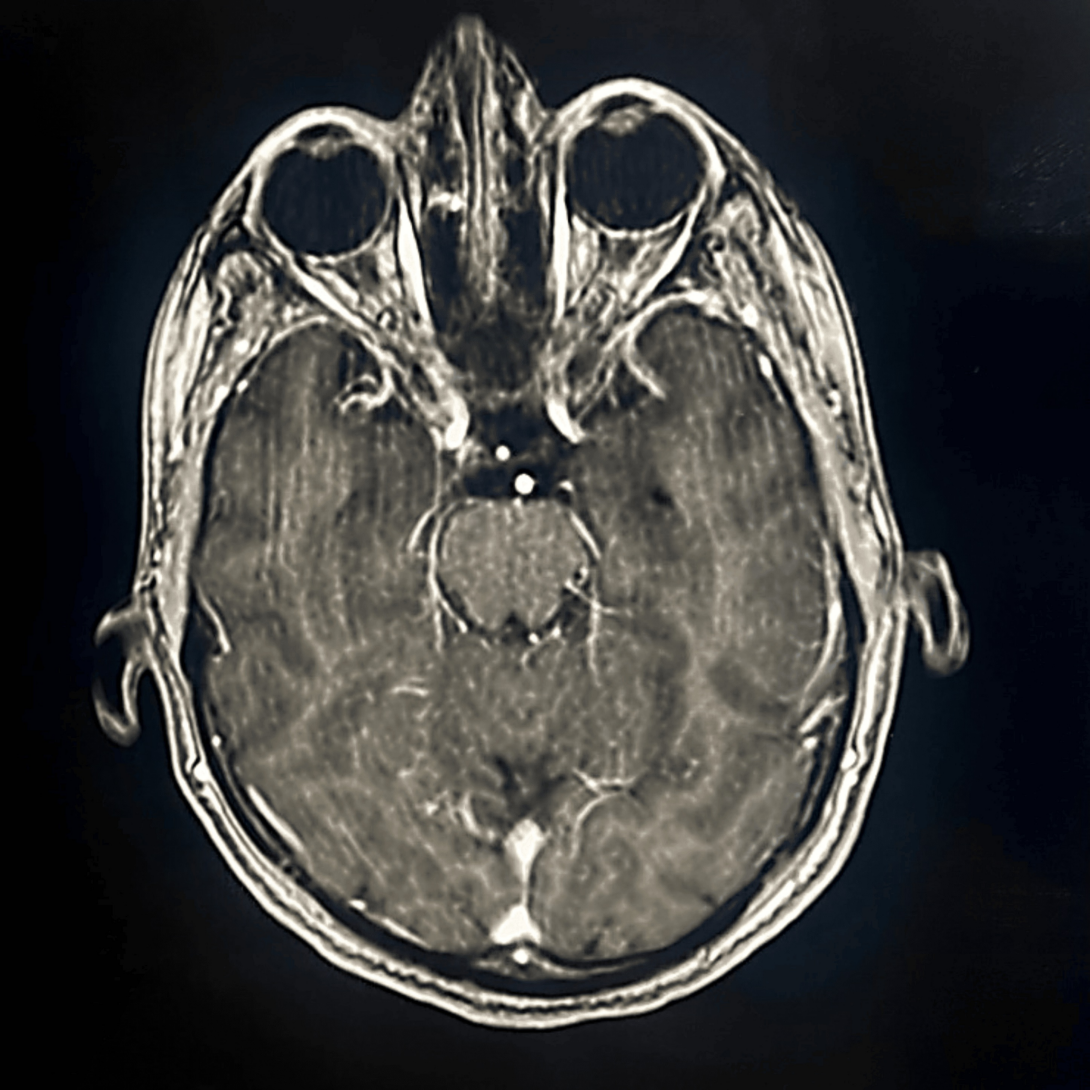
Brain MRI Both optic nerves (yellow arrows) demonstrate slight inhomogeneity and mildly increased signal intensity on both sides.
